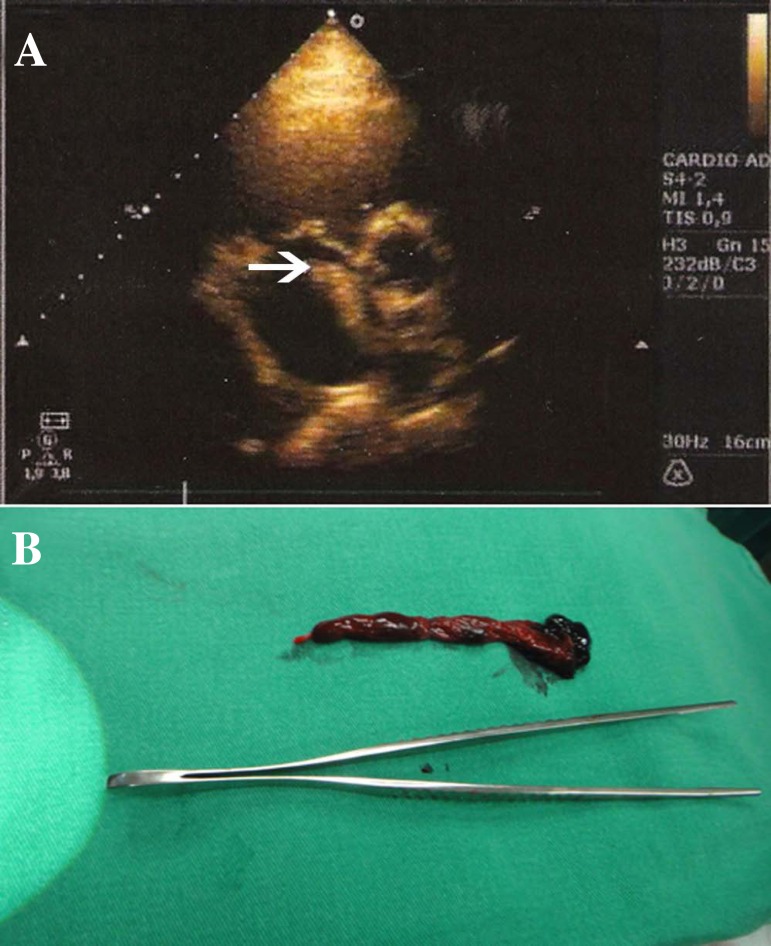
(A) Transthoracic echocardiogram showing thrombus straddling the patent
